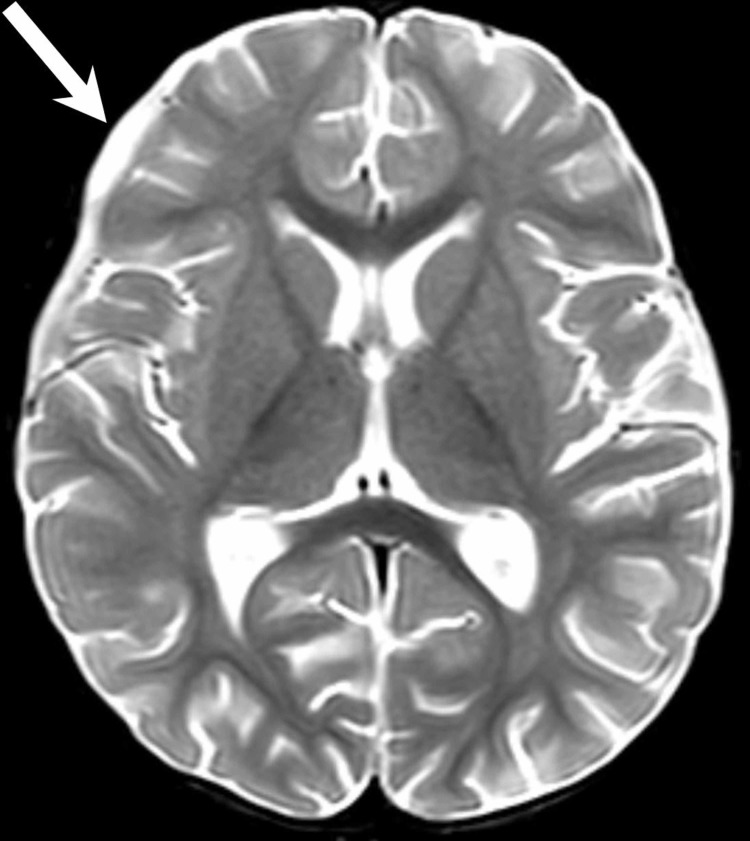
Axial, T2-weighted MRI showing bilateral subdural empyemas over the bilateral cerebral convexities most prominent in the right frontal region (arrow) which required right burr-hole craniotomy.
